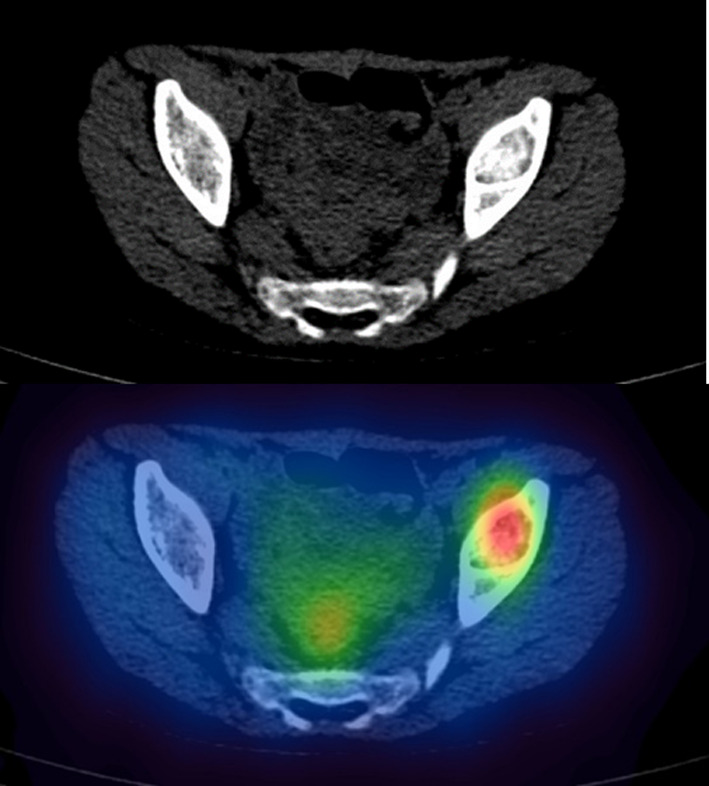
Axial CT and SPECT/CT of a bone metastases in [^131^I]mIBG patient
